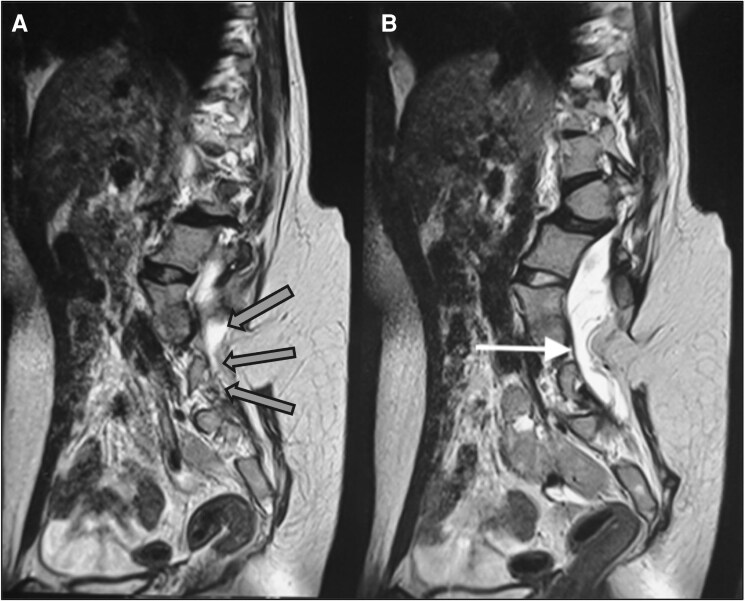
Sagittal section of magnetic resonance imaging spine in case 3 showing (A) defect in the posterior element of L5 vertebra (grey arrows) with (B) fat-containing lesion of size 2.0 × 4.0 × 3.5 cm (white arrow) in the posterior paraspinal soft tissue at the lumbosacral region communicating with the thecal sac, suggestive of spina bifida with lipomeningomyelocele.
